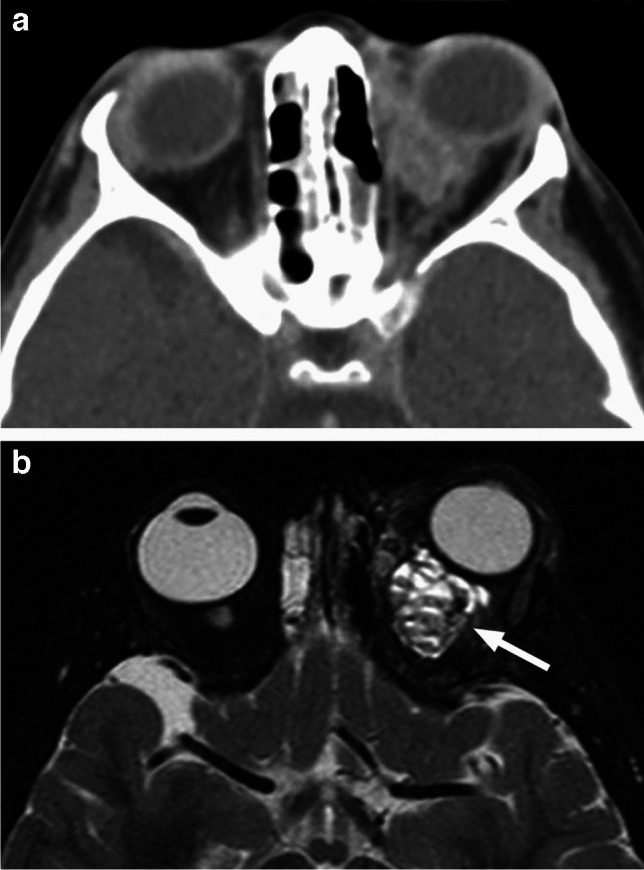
Lymphatic malformation. Axial postcontrast CT image (a) shows a heterogenous predominantly intraconal left orbital mass leading to left ocular proptosis. Corresponding T2-weighted image with fat saturation (b) reveals multicystic structure of the lesion with characteristic layering fluid levels (arrow)
